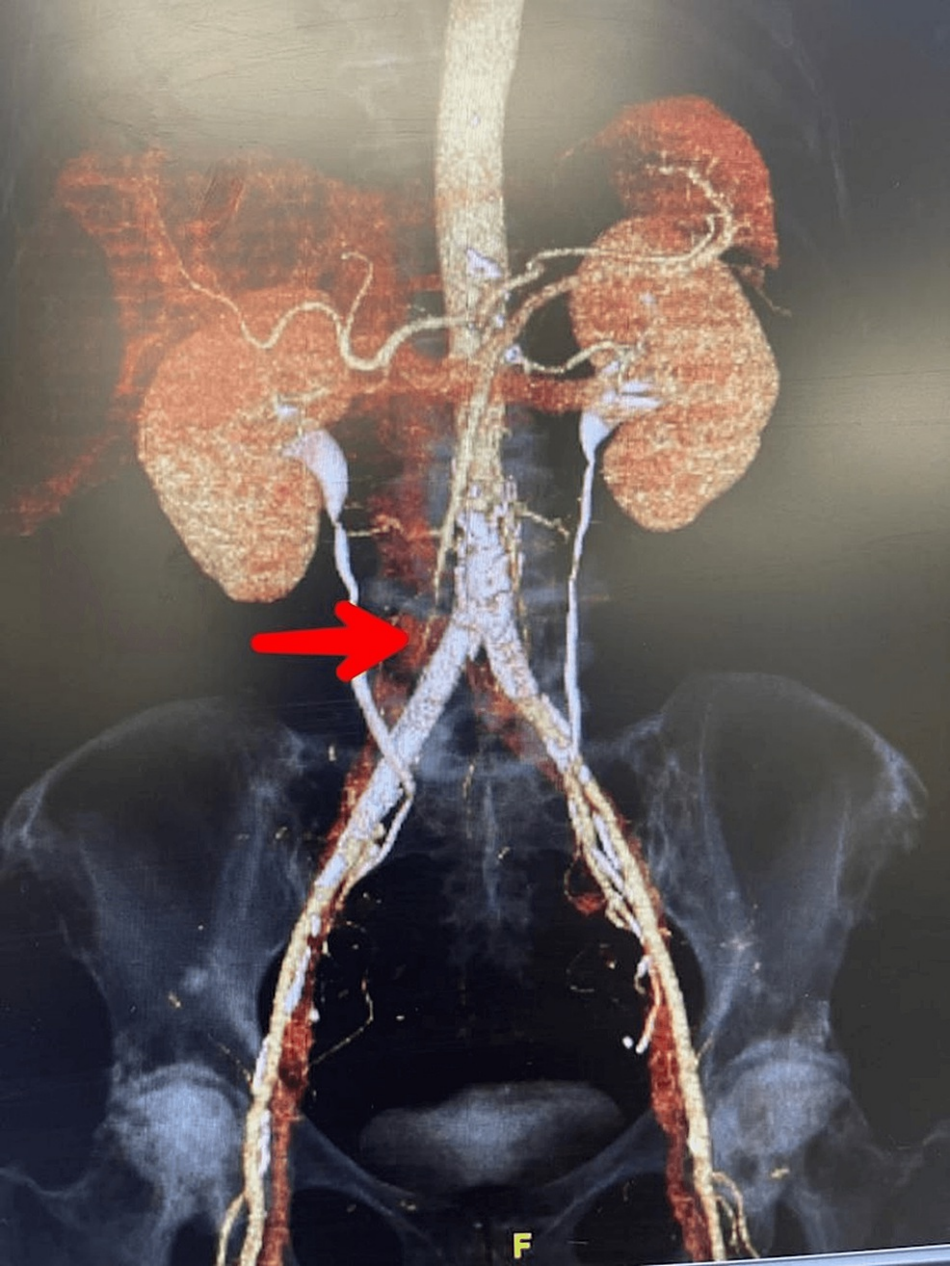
CTA, arrow pointing at diminished blood flow to the iliofemoral arteries CTA: Computed tomography angiography
